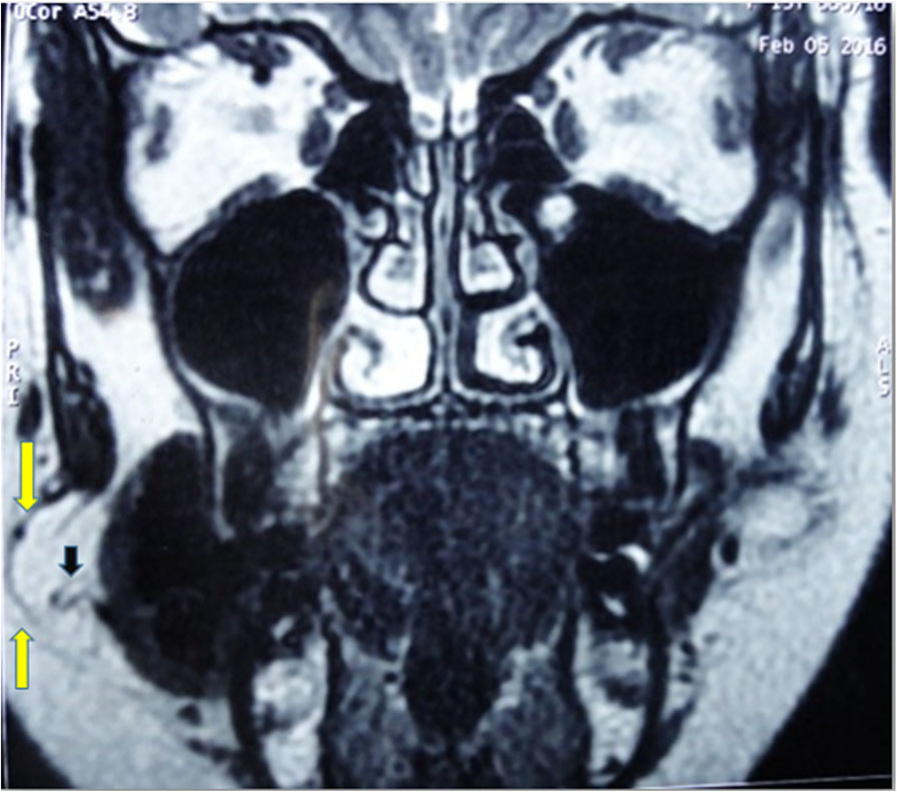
T2-weighted magnetic resonance image showing a well-defined right cheek lesion (*yellow arrows*). *Black arrow* points to the Stensen’s duct
